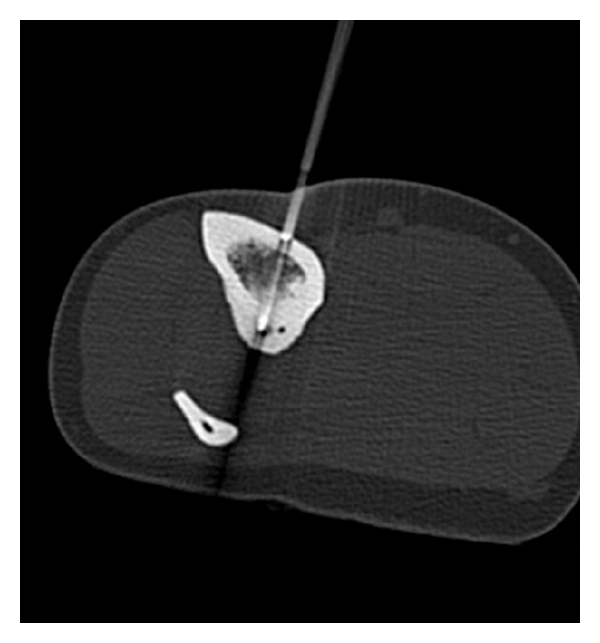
Positioning the radiofrequency probe under CT guidance.
